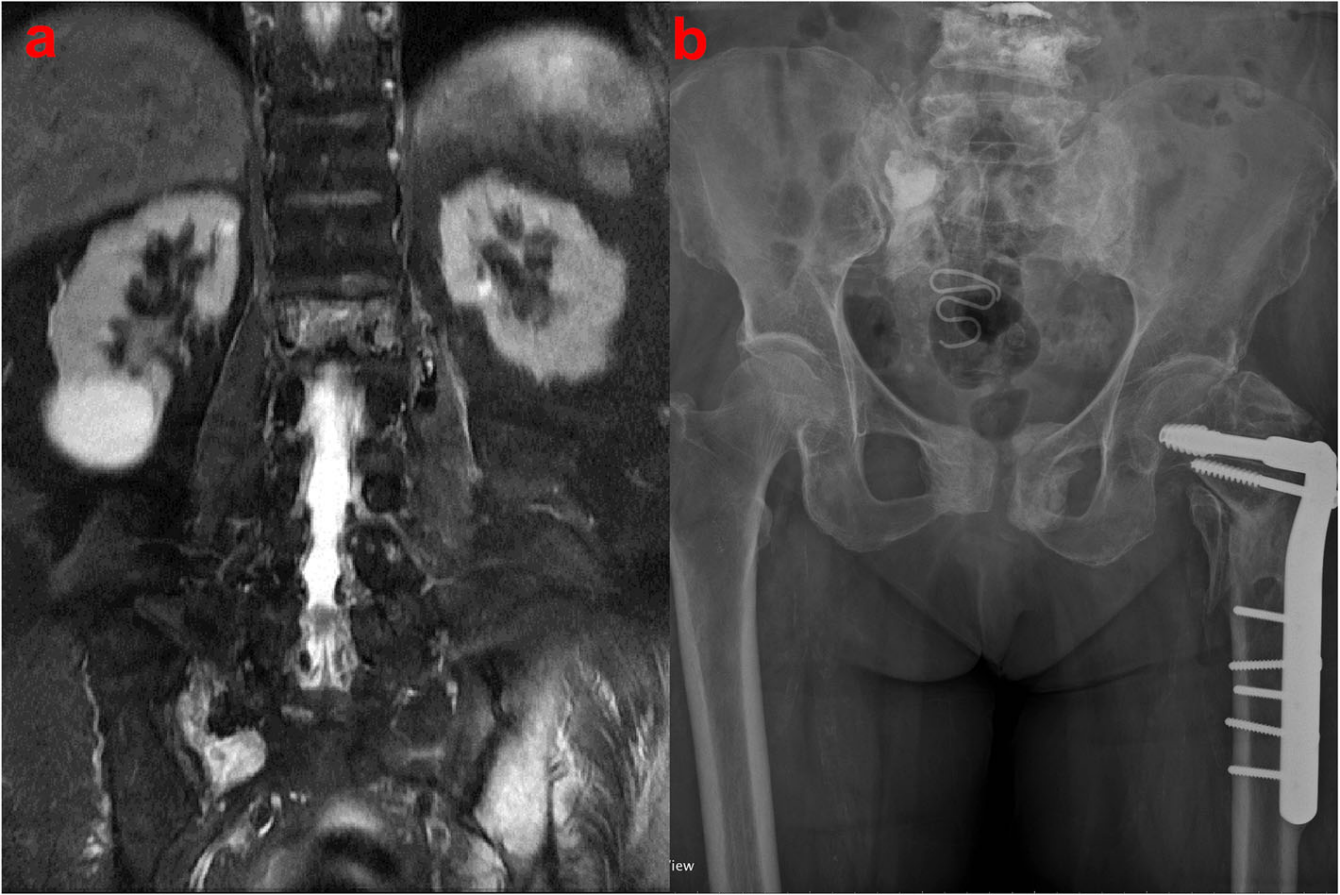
a Coronal section of spinal magnetic resonance imaging (MRI) indicating sacral insufficiency fracture involving the right alar area in an 87-year-old female patient. b Pelvis anteroposterior view depicting filling in the right alar area after sacroplasty
